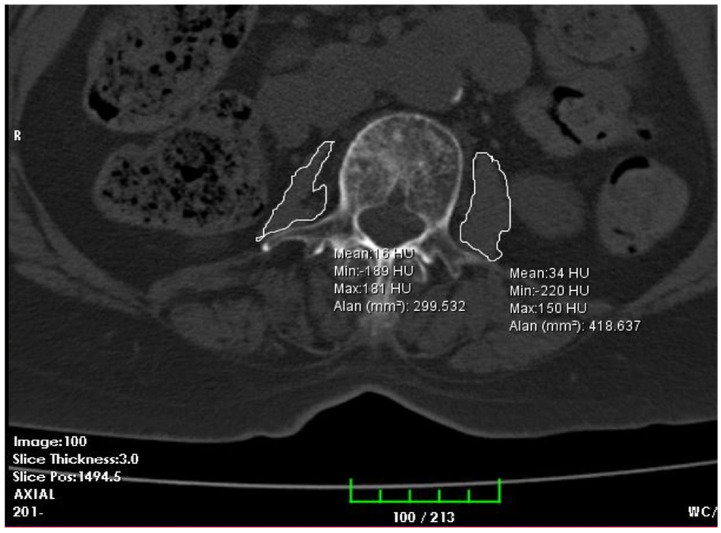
CT-based Psoas muscle measurement. Representative axial preoperative CT image at the mid-level of the L3 vertebra showing bilateral manual region of interest (ROI) delineation of the Psoas muscles. Manual boundary tracing was used to measure cross-sectional area (CSA, mm^2^) and mean muscle density (Hounsfield Units, HU) for each side, and the arithmetic mean of bilateral measurements was used for the analysis. Slice thickness: 3.0 mm.
